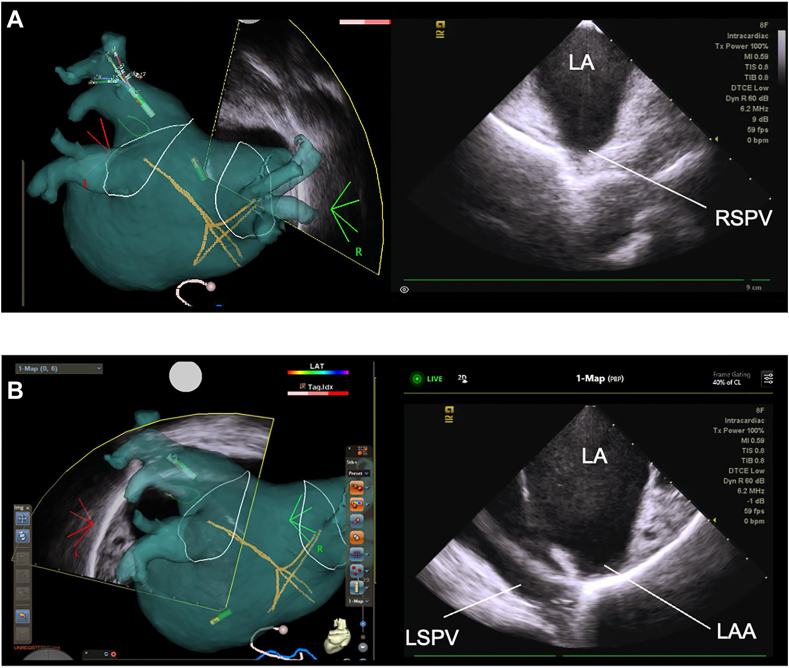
ICE catheter positioning for PV visualization during PFA. A: During right PV isolation, the ICE catheter tip was positioned centrally within the LA. By tilting the catheter anteriorly and inferiorly, the RSPV and RIPV were visualized. B: During left PV isolation, the ICE catheter tip was advanced further leftward. Posterior deflection enabled visualization of the left PVs from an inferior angle. Additional leftward and rightward deflection allowed clear visualization of the LSPV and LIPV, respectively. ICE = intracardiac echocardiography; LA = left atrium; LAA = left atrial appendage; LIPV = left inferior pulmonary vein; LSPV = left superior pulmonary vein; PFA = pulsed field ablation; PV = pulmonary vein; RIPV = right inferior pulmonary vein; RSPV = right superior pulmonary vein.
